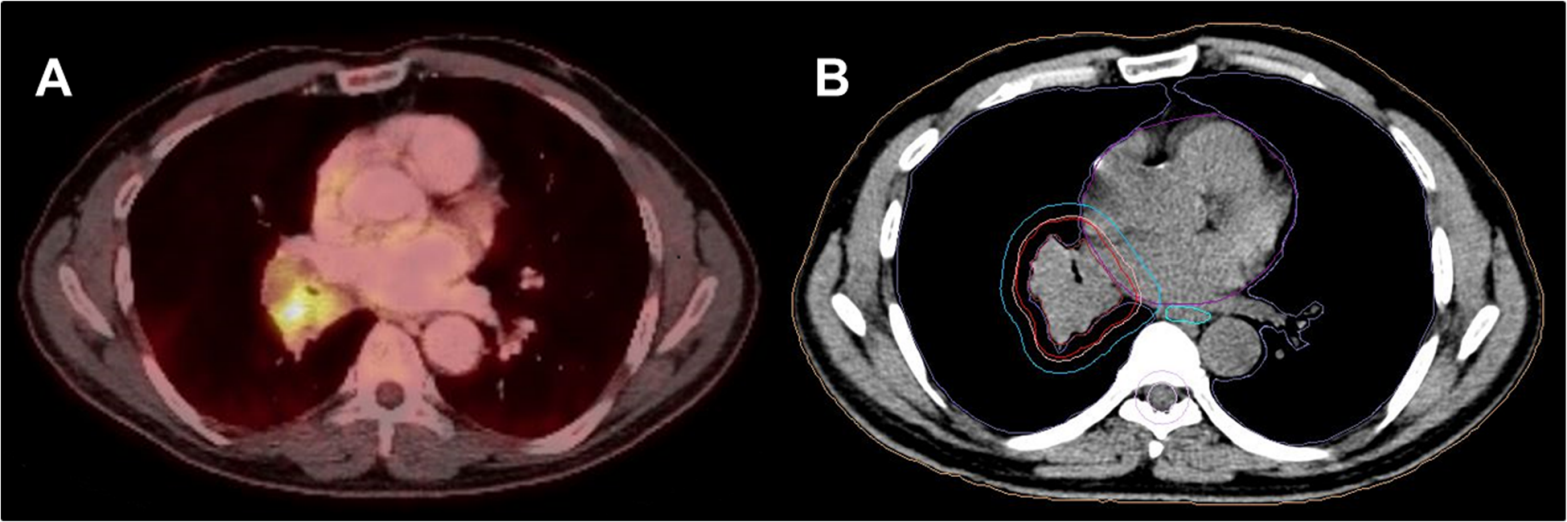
A patient with newly diagnosed NSCLC (cT2b N3 M1b) and ^18^F-FDG PET/CT for staging (a) and inclusion in radiotherapy planning (b)
